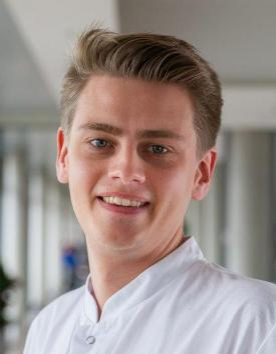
Kolja Lau, MD, began his research career in 2017 at the University Hospital Würzburg, Germany, where he focused on echocardiographic parameters in heart failure as part of his doctoral thesis. He is currently an assistant physician at one of Germany’s leading Fabry disease centres, where comprehensive patient care and the longitudinal management of this specialized patient population are central to his clinical work. Since 2021, his clinical and scientific activities have been dedicated to Fabry disease, with a particular focus on variants of uncertain significance, novel therapeutic approaches, and the diagnosis and management of Fabry cardiomyopathy.
